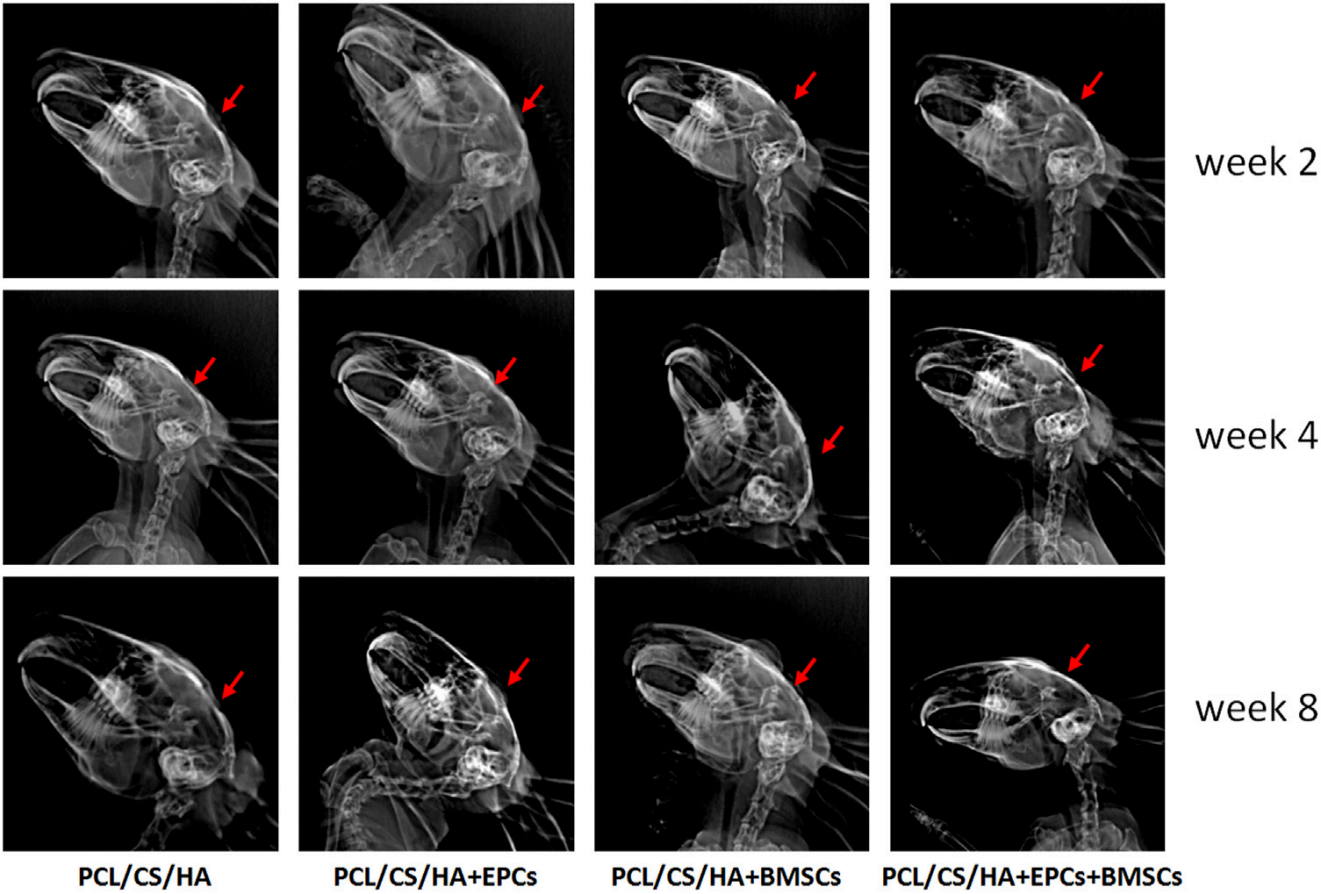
Evaluation of calvarial repair by X-ray images after 2, 4, and 8 weeks of implantation. The red arrow indicates the defect area receiving scaffold implantation.
